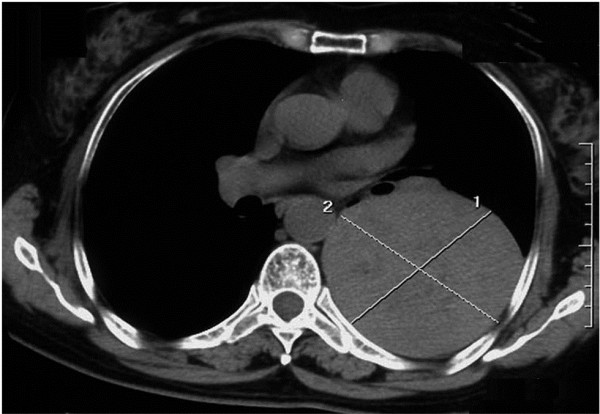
Computed tomography scan of Case 1 obtained in 2010. Computed tomography scan obtained in 2010 showing a huge soft-tissue mass with a clear periphery in the left side of the chest, measuring 8.2 cm × 8.6 cm.
